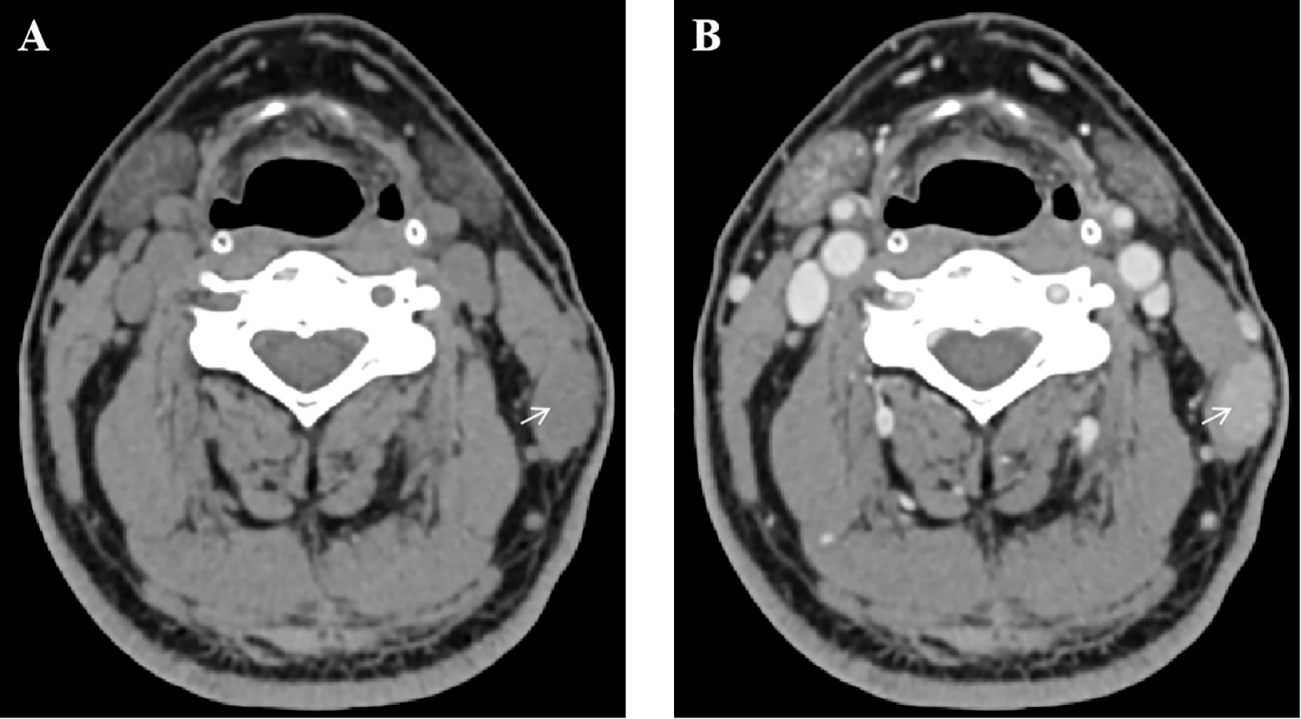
Contrast-enhanced neck CT scan demonstrating an intramuscular nodule. (A) Axial plain CT scan shows a hypodense nodule (arrow) within the left sternocleidomastoid muscle. (B) Axial contrast-enhanced CT scan in the same phase reveals progressive, uniform enhancement of the lesion (arrow).
